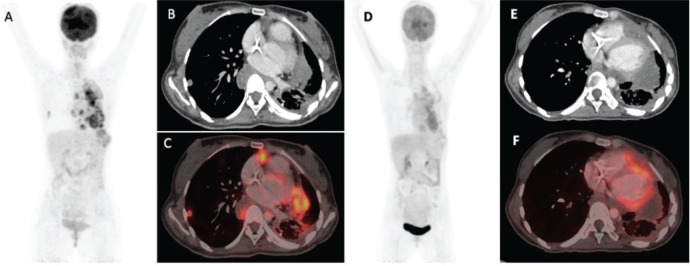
Fluorodeoxyglucose-positron emission tomography (PET) images of a patient with mediastinal SS treated with Anlotinib. (a): Pre-anlotinib maximum intensity projection (MIP), trans-axial computer tomography (CT) (b): And fused PET/CT (c): Images showing multiple variable sized lung subpleural and parenchymal nodules with some showing necrotic changes in bilateral lungs. (d–f): Post-anlotinib images showing decrease in size, uptake and number of most of the parenchymal and subpleural lesions in bilateral lungs with increase in associated necrotic component.
